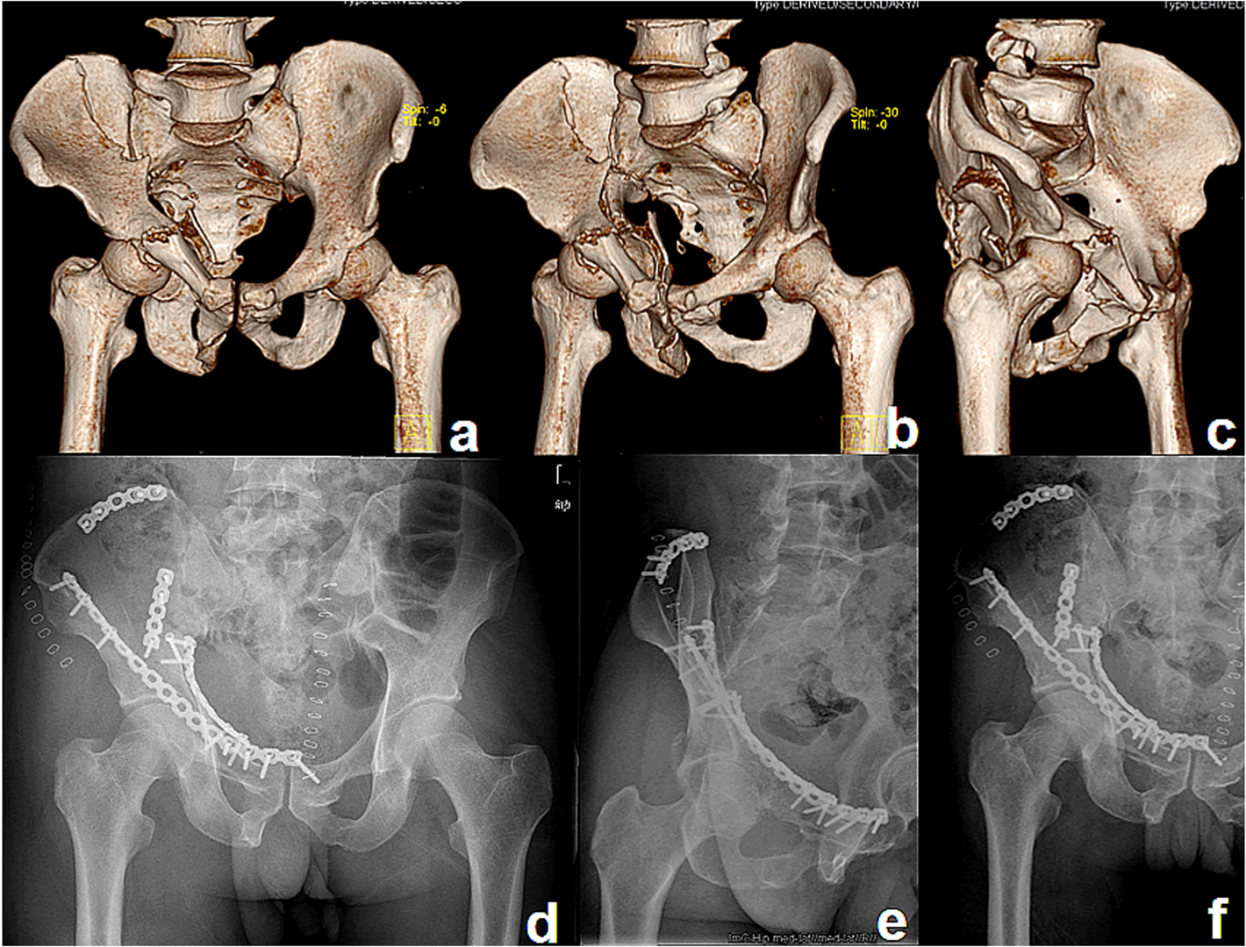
A patient treated with the S+IF approach. Preoperative 3D computed tomography reconstructions (a–c) of a both-column fracture showing the severity of fracture displacement. Postoperative radiographs (d–f) showing good reduction and fixation
